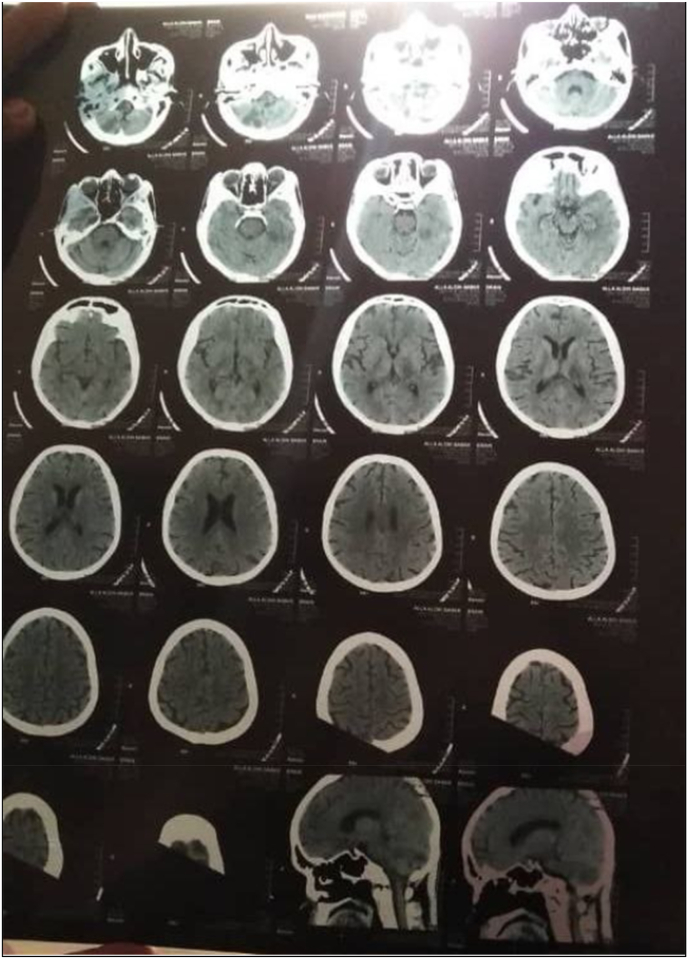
Brain CT Scan which showed left parietal area infarction.
